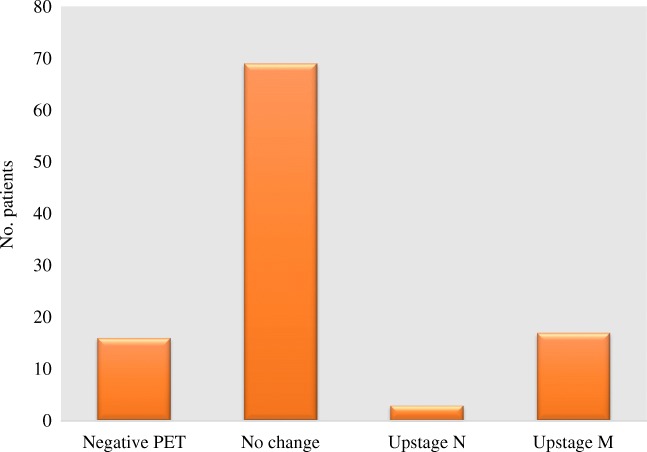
Change in TNM stage following PET-CT (*n* = 105). Negative PET = 16 patients; no change = 69 patients; upstage N = 3 patients; upstage M = 17 patients
